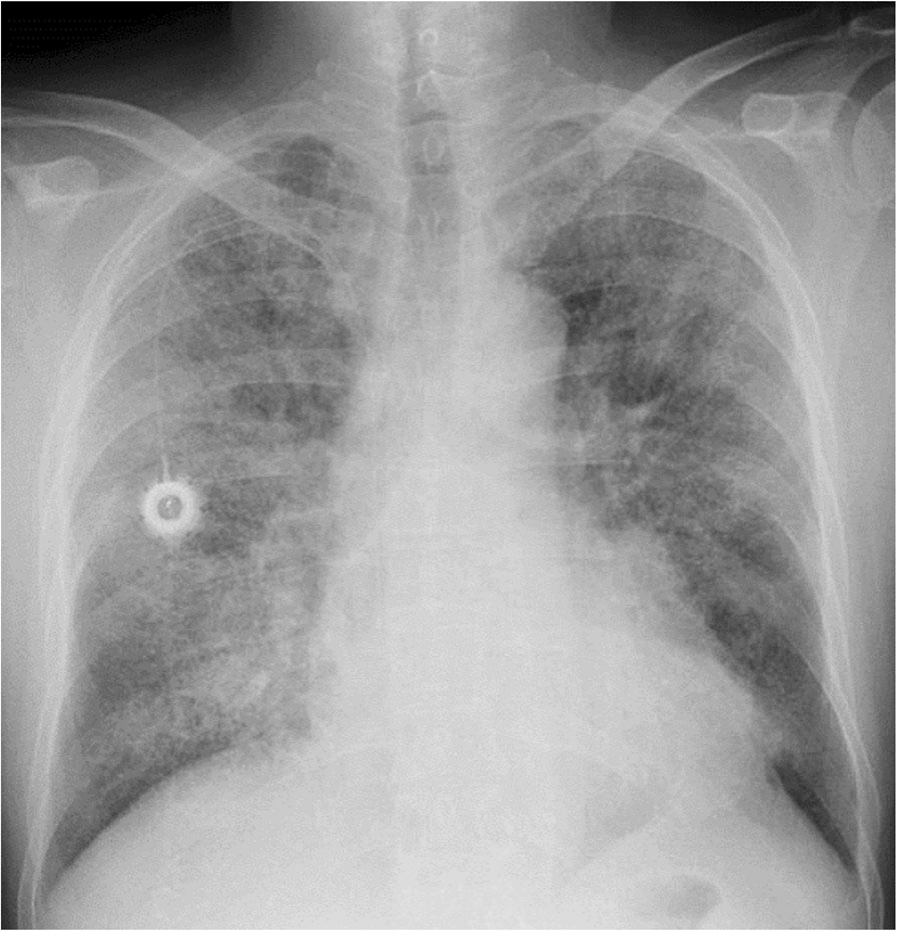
Chest X-ray obtained in June 2014 showing reticulonodular shadows in both lung fields
